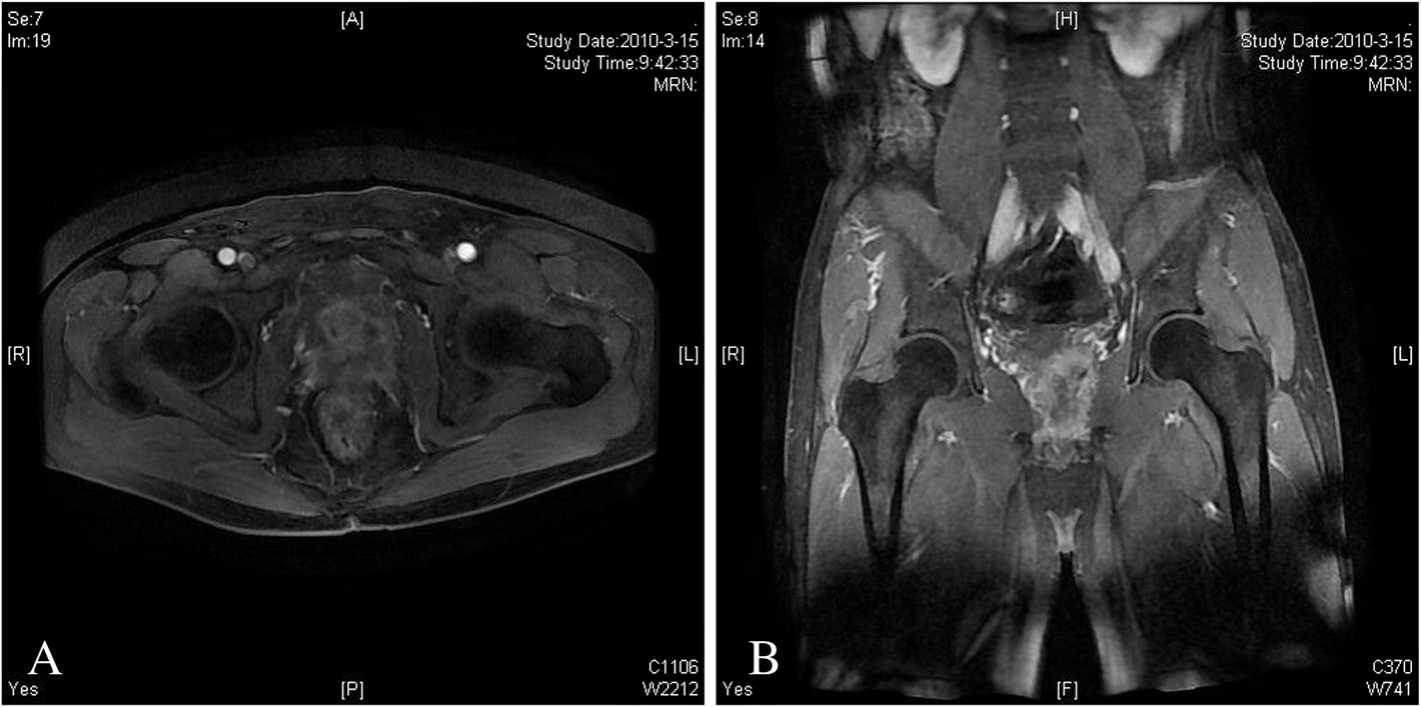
Magnetic resonance imaging: Both the axial (A) and the coronal (B) images suggested extra prostatic extension and the invasion of the bladder wall and the rectum.
